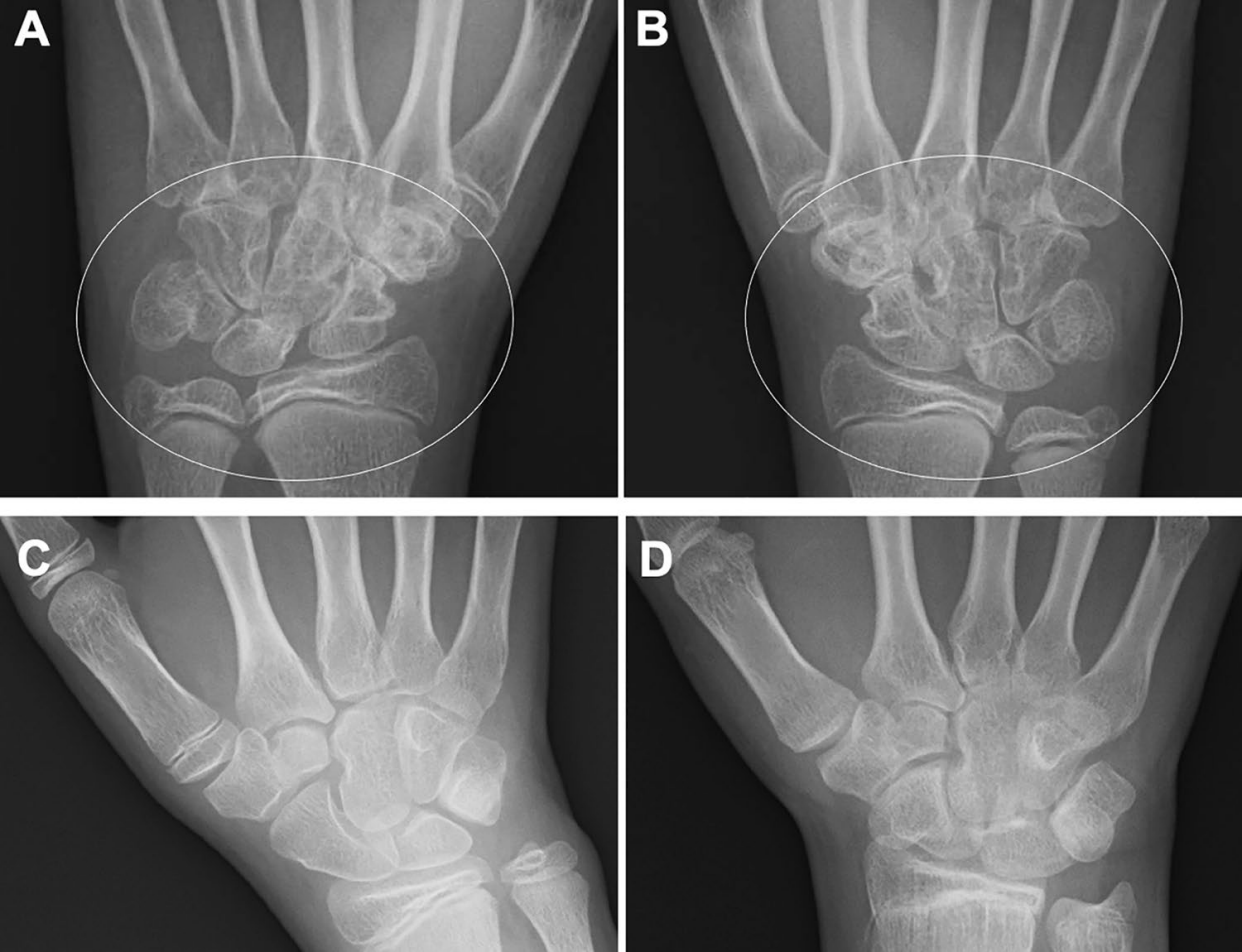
Two cases with RF positive polyarticular type JIA. A–B. A 14-year-old girl. The frontal radiographs of the hands showed bilateral, symmetric carpal erosions and carpal joint space narrowing (circles, A, B). C–D. A different girl. The radiographs showed progression of joint space narrowing and development of carpal erosion between age 8 years (C) and 12 years (D)
